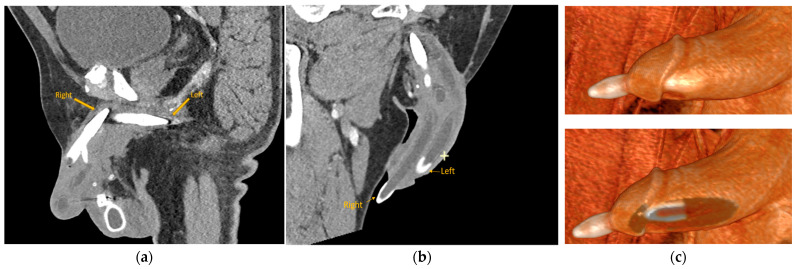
Contrast-enhanced CT of the abdomen and pelvis. (a) Sagittal reformation demonstrating the anterior displacement of the right metallic rear tip extender and the normal position of the left rear tip extender. (b) Oblique reformation of the penis demonstrating the transurethral extension of the right penile cylinder. (c) Volumetric 3D rendering of the penile prosthesis demonstrating the abnormal protrusion of the right penile prosthesis cylinder through the meatus, and left cylinder in the expected location.
